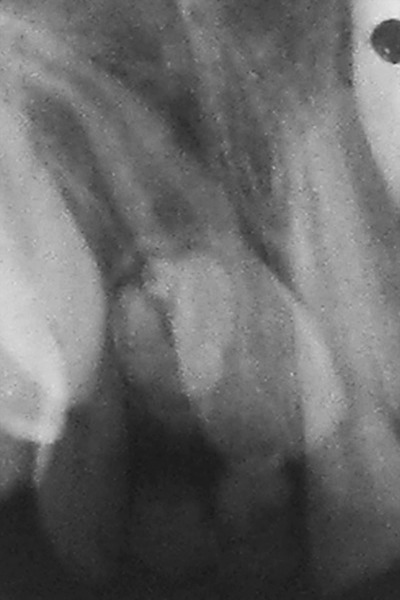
Periapical radiograph showing a single lesion of compound odontoma
